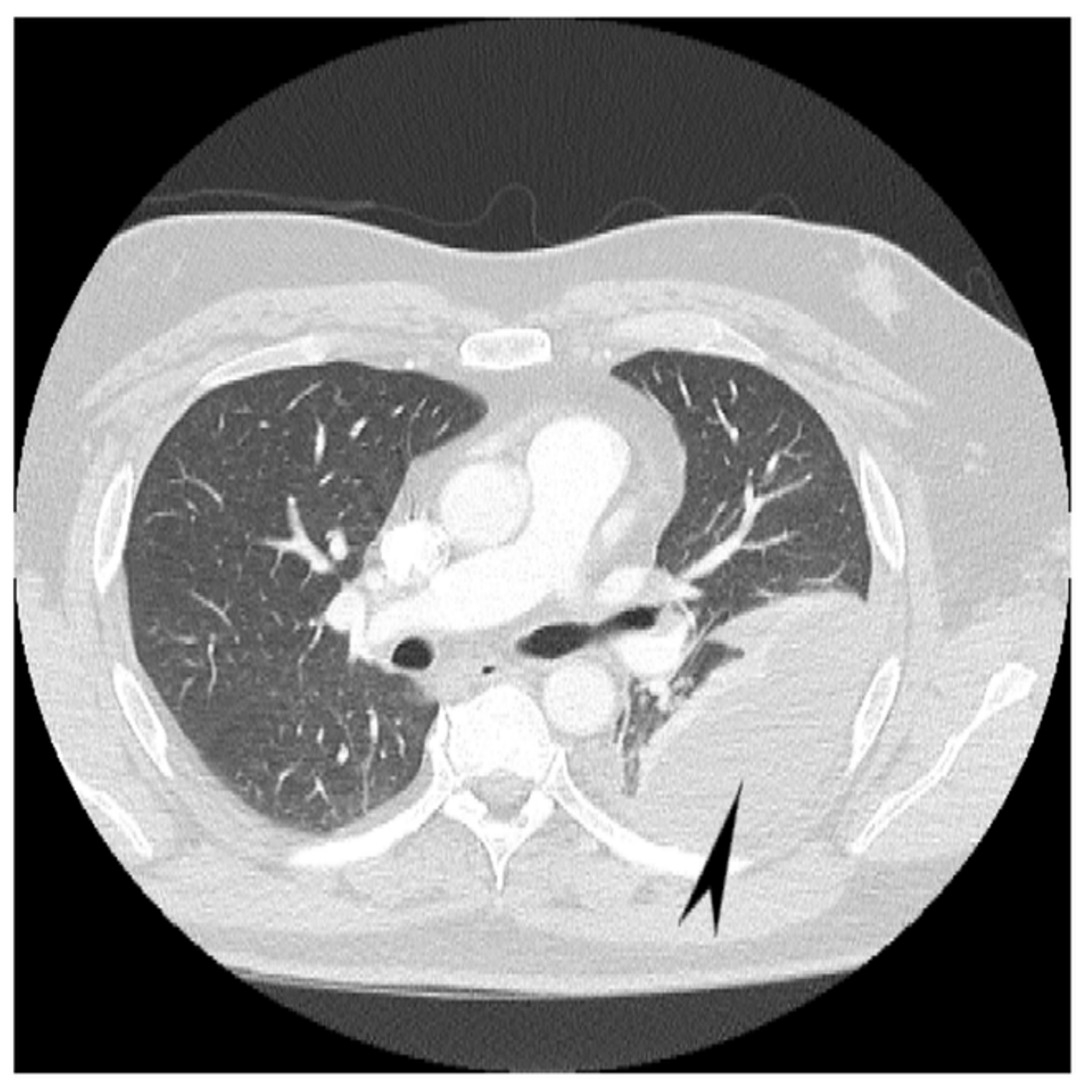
CT of the chest reveals a small-to-moderate loculated left pleural effusion with left basilar atelectasis (arrowhead) There is no CT evidence of pulmonary embolism CT: computed tomography
